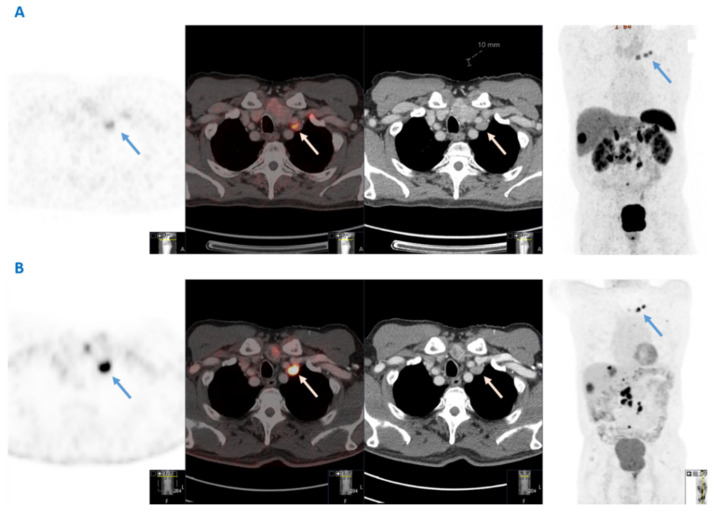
Dual functional imaging of a patient with a G2 small intestinal neuroendocrine neoplasm and Virchow’s node metastases (blue and white arrows), both ^68^Ga-DOTATATE- and ^18^F-FDG avid. (A) From left to right: ^68^Ga-DOTATATE PET; fusion; computed tomography; and maximum intensity projection (MIP) images (B) From left to right: ^18^F-FDG PET; fusion; computed tomography; and maximum intensity projection (MIP) images.
